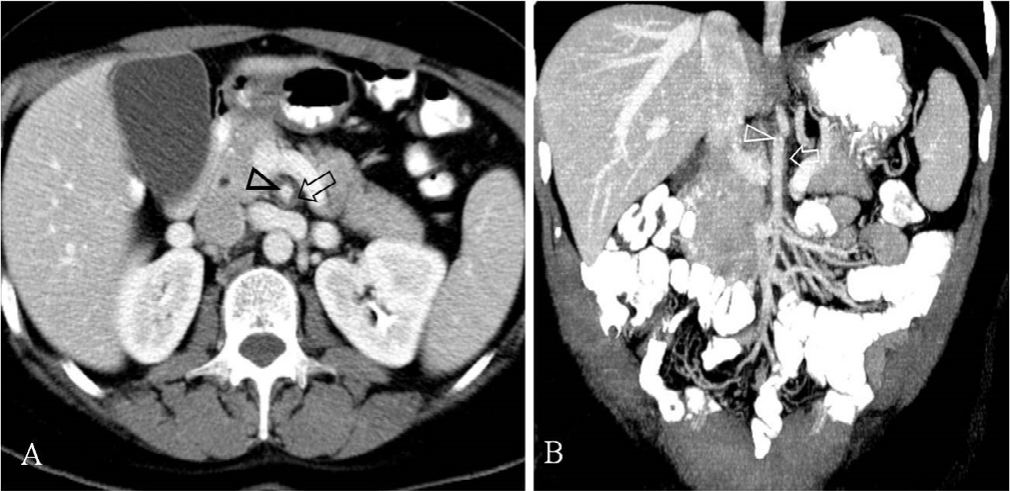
CT scan following intravenous contrast administration, split-bolus excretory-portal phase. (A) Axial slice shows the superior mesenteric artery containing a hypoenhancing line (arrowhead) and surrounded by perivascular fat stranding (arrow). (B) Coronal reconstruction shows a short line at the upper aspect of the lumen (arrowhead) and thickening of the adjacent wall (arrow). Findings were initially interpreted as dissection.
